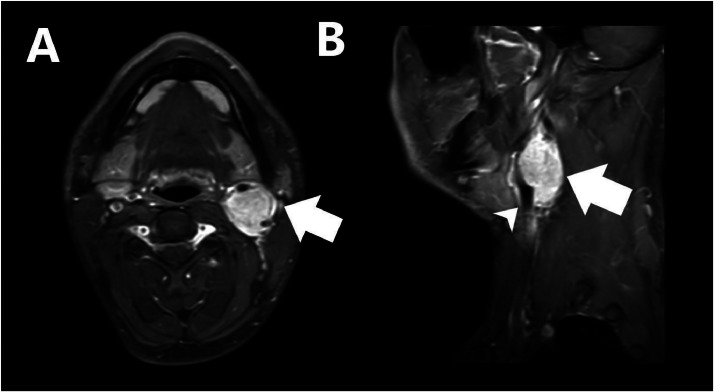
T1 Contast Axial (A) and Sagittal (B) MRI neck showing enhancing mass lesion (arrow) in left carotid triangle at the left common carotid artery bifurcation (arrowhead) causing splaying of ECA and ICA. Legend: T1 contrast, T1 weighted image contrast; MRI, Magnetic resonance imaging; ICA, Internal carotid artery; ECA, External carotid artery.
